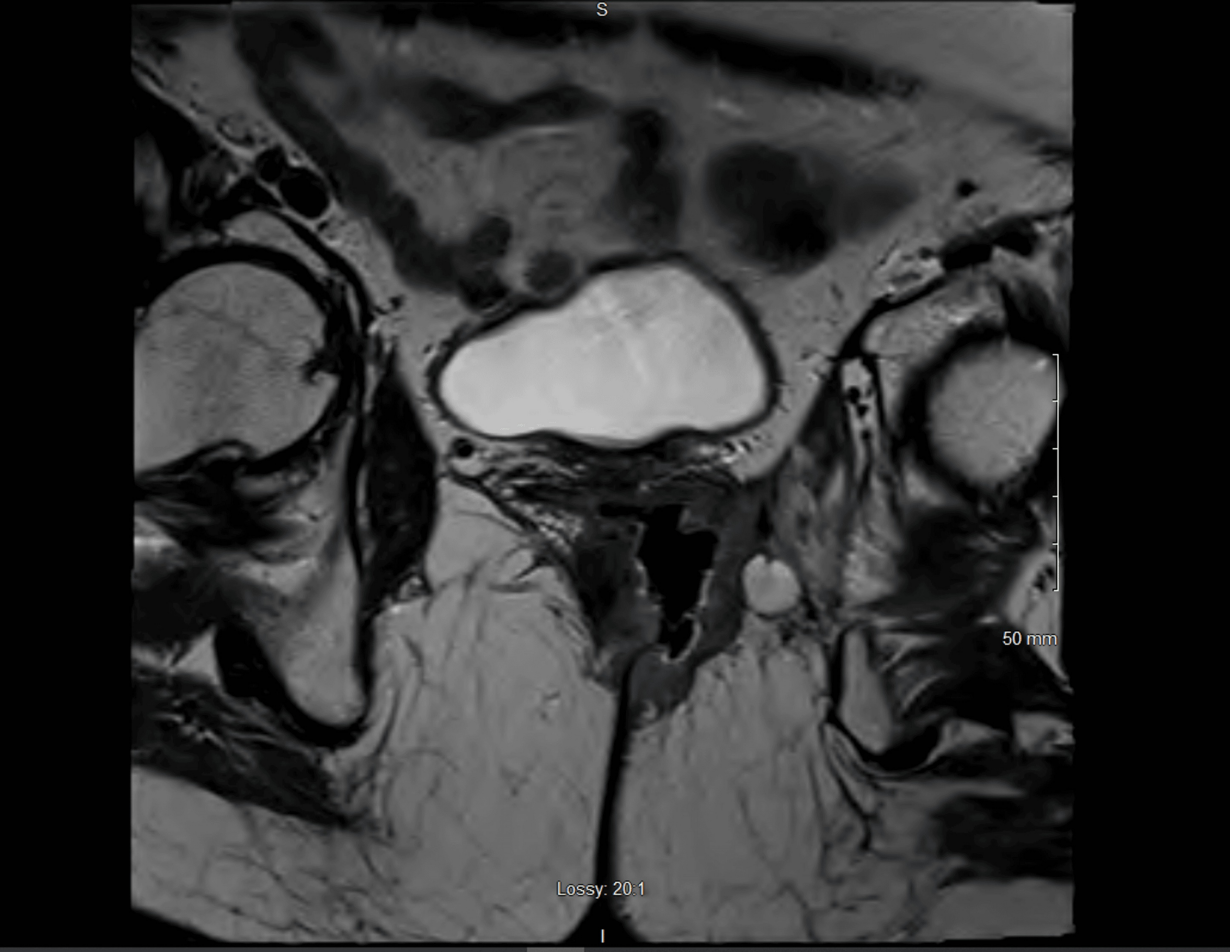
Pelvic MRI showing locally advanced recurrent anal cancer in the axial planes for case 2
